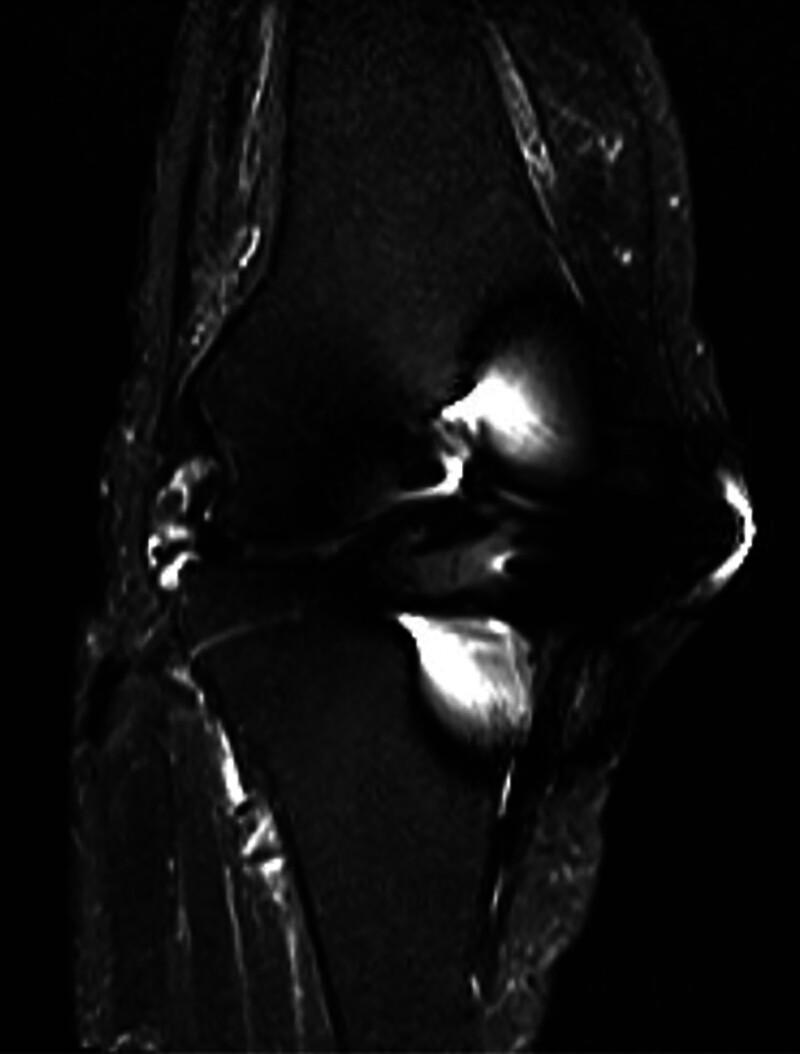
The coronal view of T2-fat suppression MRI for the right knee joint after steroid administration.
